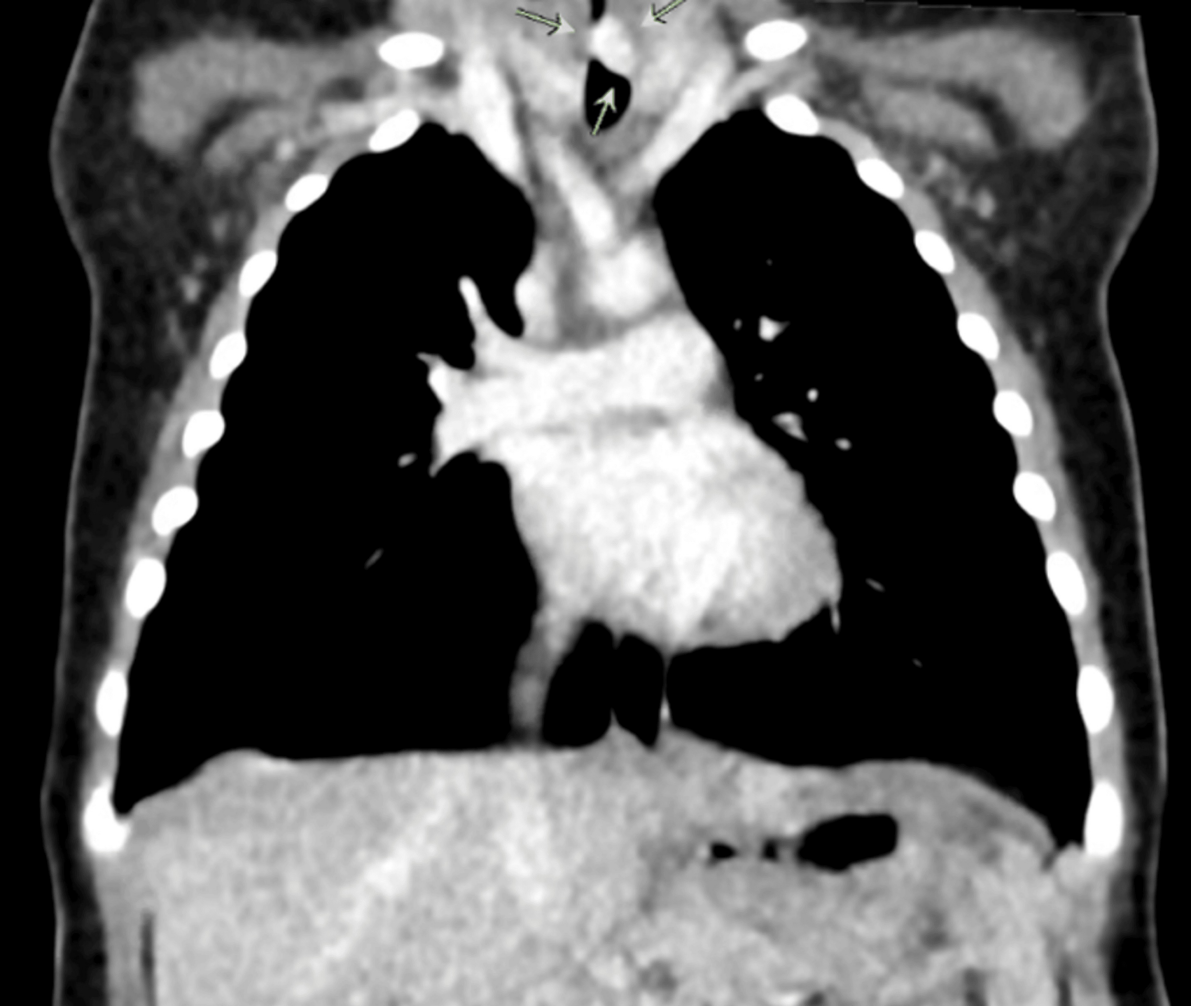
Coronal CT angiogram showing a soft-tissue mass compressing the proximal airway (shown by arrows). CT: computed tomography.
